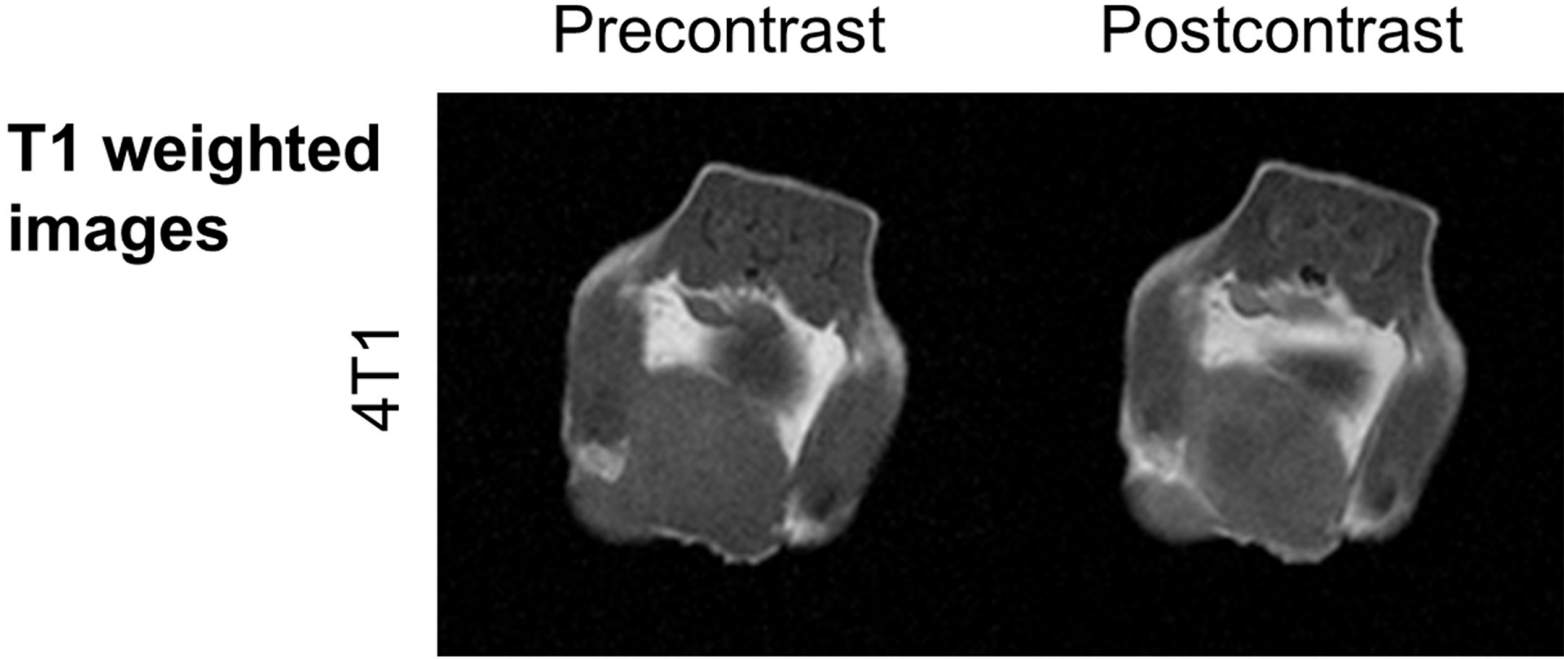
Gadopentetate does not delineate early necrosis. Axial T1 weighted MR images of the same 4T1 tumor as in Fig 1 at 60 seconds after intravenous injection of Gd-DTPA at a dose of 0.2 mmol/kg. Note homogenous tumor morphology with only slightly increased enhancement of the tumor periphery compared to the tumor center.
